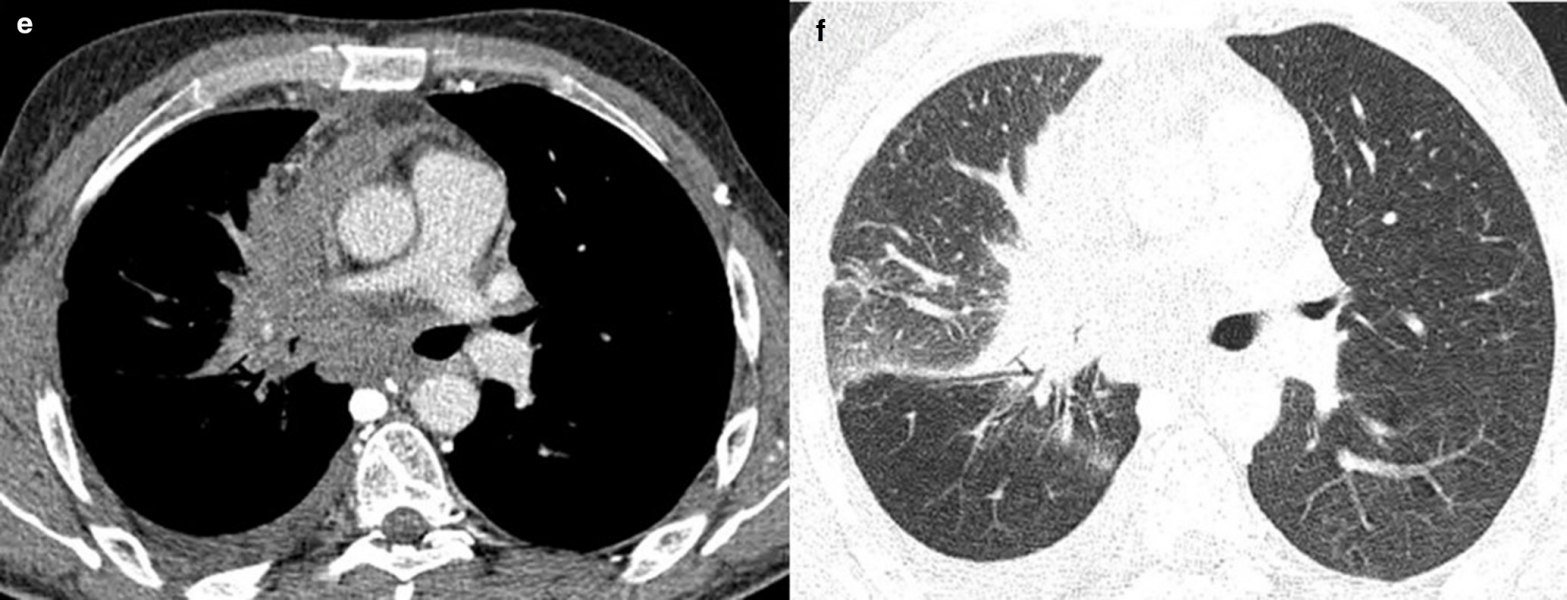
(E-F) Contrast-enhanced chest CT scans in mediastinal (E) and lung window (F) showed mildly enhancing irregular right hilar mass (arrow).
